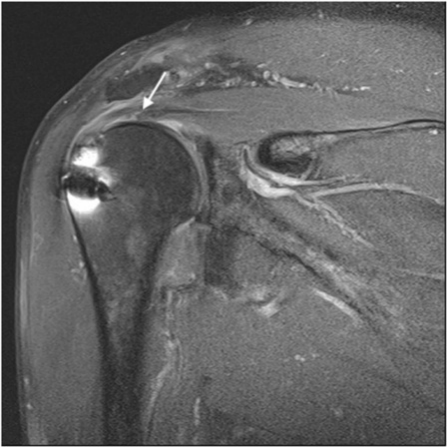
A postoperative MRI of a Sugaya type III result shows deep layer re-tear (white arrow)
